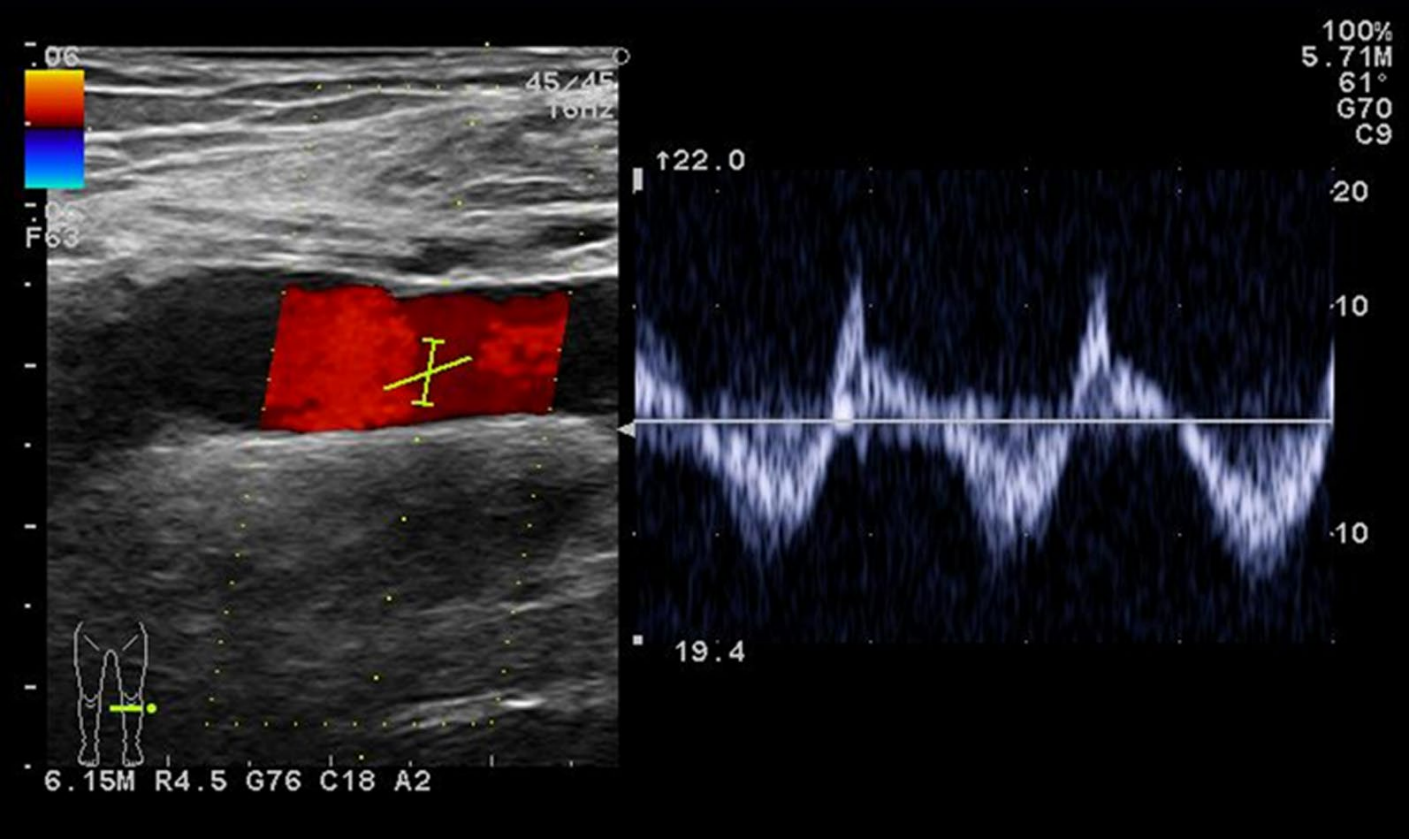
Ultrasonography showed pulsatile regurgitation in the left GSV
